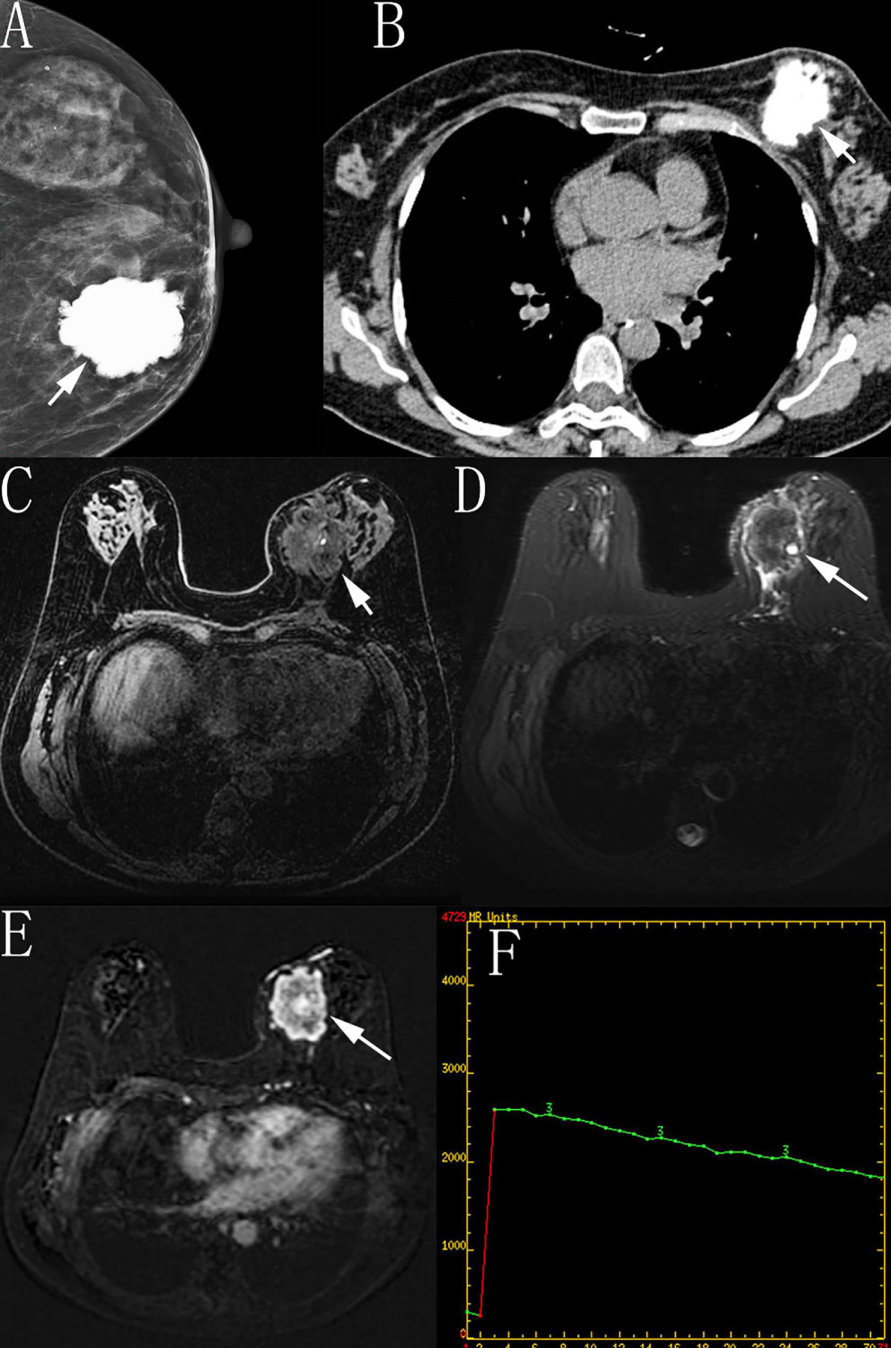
(A-F) Mammography and chest CT showed a hyperdense mass with lobulated borders (A, B). On fat-suppressed T1-weighted image, the tumor was hypointense or isointense, while on fat-suppressed T2-weighted image it was mildly hyperintense and hypointense along with circular hyperintensity (C, D). Contrast-enhanced MRI showed uneven enhancement, and the time-intensity curve on DCE-MRI was of rapid uptake and slow reduction type (E, F).
